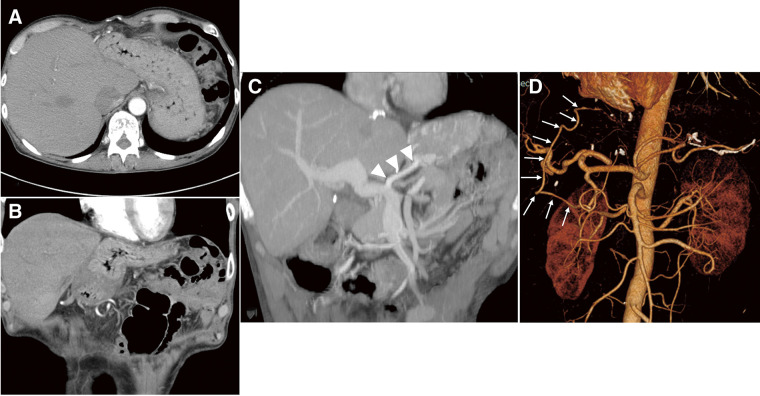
CT findings. (A) Axial image and (B) coronal image: Extensive contact between the anterior wall of the stomach and the liver. (C) Coronal image. Dilatation of the coronary vein (arrowheads). (D) Three-dimensional (3D) CT angiography. The right gastroepiploic artery is displaced cranially (arrows). CT: computed tomography
